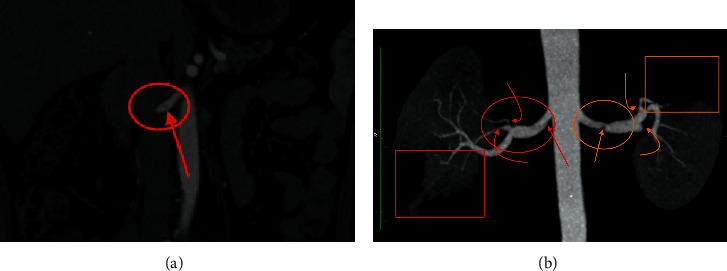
(a) Coronal CT angiography image and (b) anterior volume-rendered 3D MPR coronal image were obtained after intravenous contrast material in arterial phase reveals dissection flap (straight red arrow) of the right renal artery (red circle) in (a); (b) demonstrates focal eccentric thrombosis and attenuated flow and reduced caliber of the lower lobe segmental branch (curved red arrow) and upper lobe subsegmental branch (angled red arrow), resulting in infarct (red rectangular); and also demonstrates focal thrombosis in the mid-left main renal artery and dissection flap (orange circle), causing significant stenosis (orange straight arrow) and complete thrombosis of the middle segmental branch of the left renal artery (curved orange arrow) and partial stenosis of the upper segmental branch (angled orange arrow) with new development of infarct in some part of the mid pole as well (orange rectangular).
